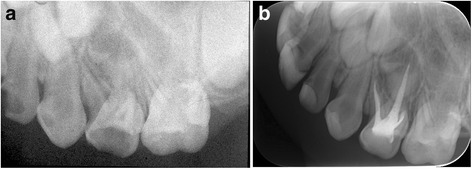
Eighteen months follow-up of MPRCF. a Pre–operative radiograph of maxillary first left primary molar of a 3-year-old girl considered for root canal treatment with MPRCF b Radiograph taken 18 months postoperatively showing no resorption of materials in all roots canal
